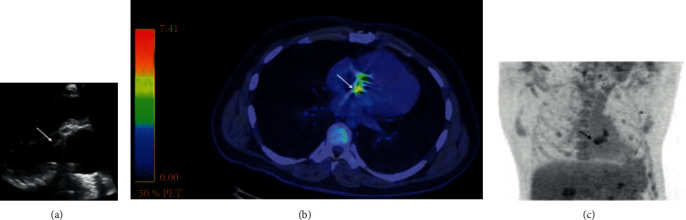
Transesophageal echocardiography image of patient 1 (panel (a)); arrow shows vegetation. ^18^F-FDG-PET/CT images of patient 1 (panels (b) and (c)). Arrows show increased ^18^F-FDG uptake at the site of aortic valve prosthesis.
